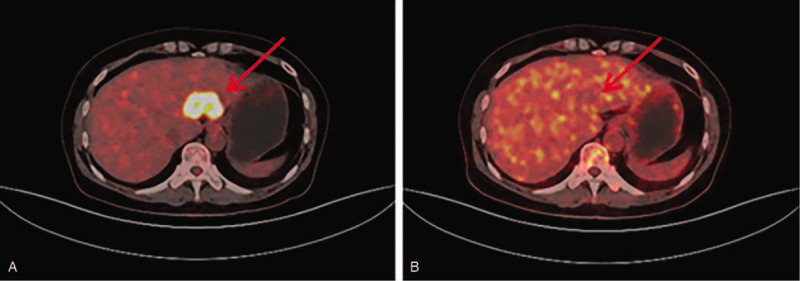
PET-CT showed metastasis in the left liver. (A) Before treatment with pembrolizumab and nab-paclitaxel, the metastasis was 4 cm in diameter. (As indicated by the red arrow, the same below). (B) After 6 cycles of pembrolizumab and nab-paclitaxel, the metastasis was nearly dismissed. PET-CT = positron emission tomography-computed tomography.
